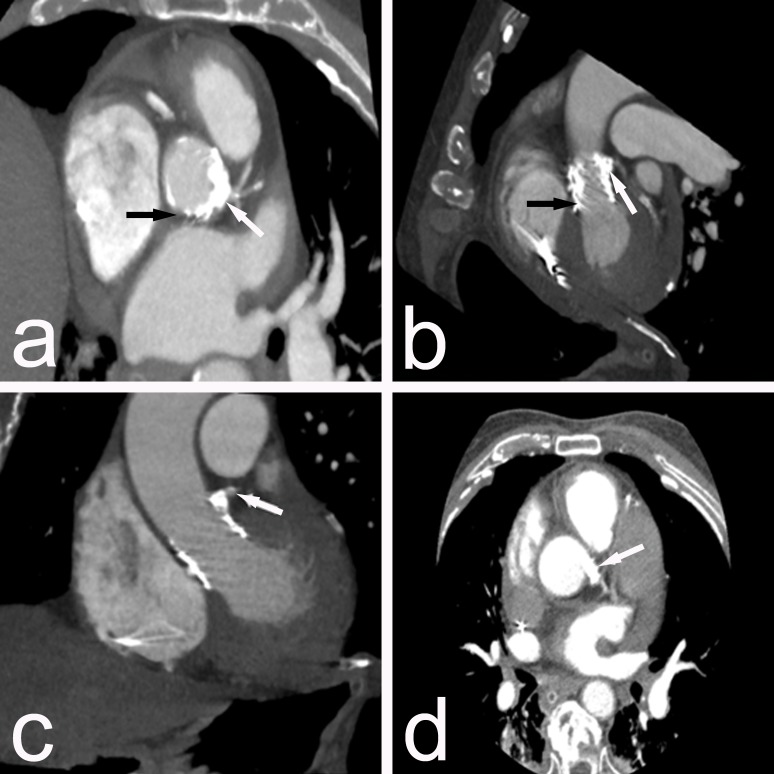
Control multidetector CT. a, b) showing Edwards Sapien 3 valve (black arrows) and protrusion of calcified valve leaflets in the left coronary sinus (white arrows); c, d) patient stent in LMCA (white arrows). CT - computed tomography, LMCA - left main coronary artery
